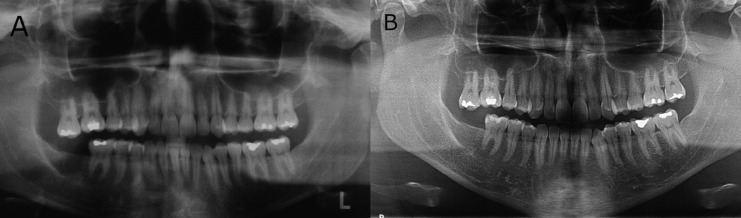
A) Panoramic radiography showing aspect of normality in the area repair process after 7-year follow-up. B) Panoramic radiography showing aspect of normality in the area repair process after 13-year follow-up.
